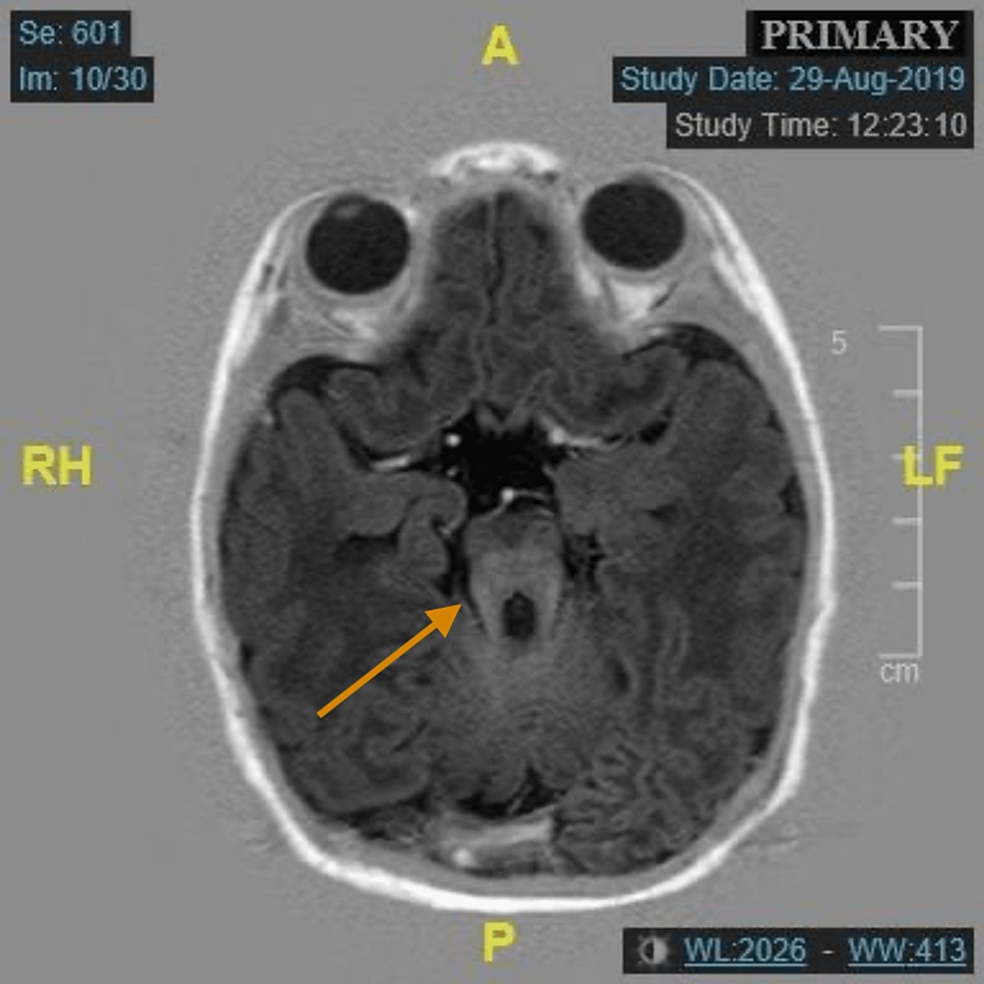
Axial section of a T1-weighted MRI showing the pathognomonic molar tooth sign (orange arrow). MRI: magnetic resonance imaging, A: anterior, P: posterior, RH: right, LF: left, CM: centimeter
